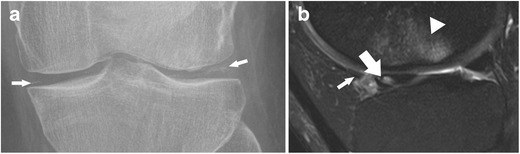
Standard radiograph (a) of the right knee in a 42-year-old male patient with a mild knee trauma shows typical signs of chondrocalcinosis with calcification (*small arrows*) within the articular space. Sagittal T2 weighted fat-suppressed fast spin echo image (b) shows traumatic bone marrow abnormalities (*arrowhead*) within the lateral femoral condyle and an oblique tear of the root of the anterior horn of the lateral meniscus (*arrow*). The tear should not be misinterpreted as the anterior transverse ligament or the geniculate ligament (*small arrow*), which is relatively thin, but intact in this patient
